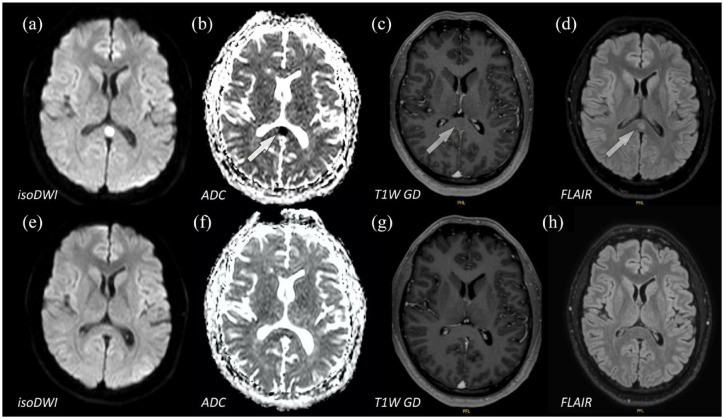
(a–d) Showing brain MRI on day 2, (e and f) follow-up MRI on day 13. On
